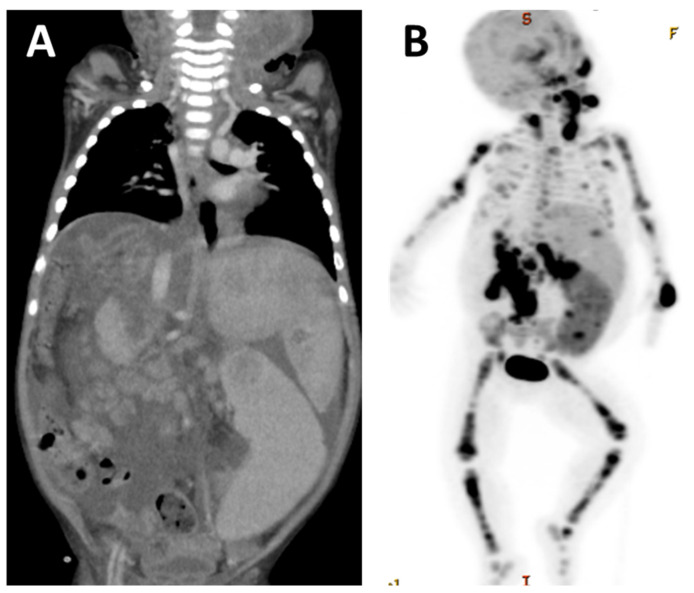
Monomorphic PTLD; PTL-NOS. Case 3. A girl of 1 year and 6 months of age. She received LDLT at 11 months of age due to biliary atresia. At 7 months after surgery, she was admitted to the hospital due to fever and enlargement of her left posterior cervical lymph nodes. (A) The initial CT scan revealed left cervical lymphadenopathy and multiple enlarged abdominal lymph nodes, and mass formation was observed in the spleen. (B) PET-CT showed the diffuse accumulation of radionuclides in the lymph nodes, bones, and spleen.
